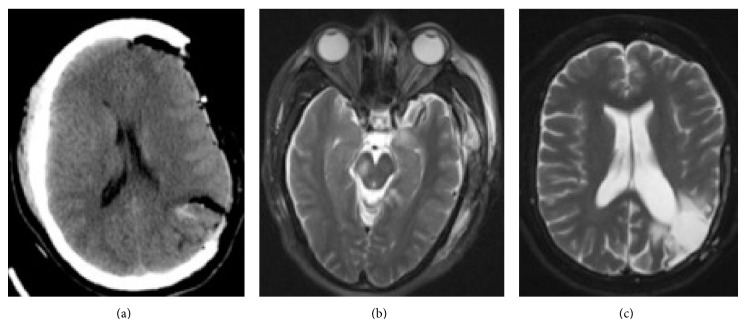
(a) Axial CT status after craniectomy, demonstrating partial clot evacuation and improved midline shift. The AVM is still present with residual hemorrhage; (b) axial T2 MRI postoperative day 2 demonstrating ischemic damage in the left hippocampus and right cerebral peduncle in the area of the corticospinal tract (Kernohan's Notch); (c) axial T2 MRI demonstrating complete resection of the AVM and cranioplasty.
